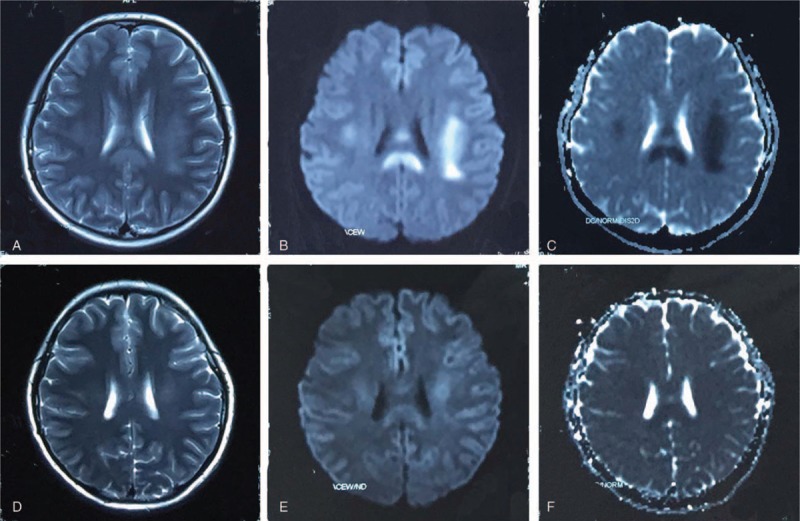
MRI imaging at 24 hours showing increased signals on T2-images in the SCC and frontal-parietal subcortex of both cerebral hemispheres (A). Abnormal signals were also found at DWI (B) and ADC images (C) in the same area. MRI imaging at 10 days later exhibited reduced abnormal signals in T2 (D), DWI (E), and ADC (F) in the same area. ADC = apparent diffusion coefficient, DWI = diffusion-weighted imaging, MRI = magnetic resonance imaging, SCC = splenium of the corpus callosum.
